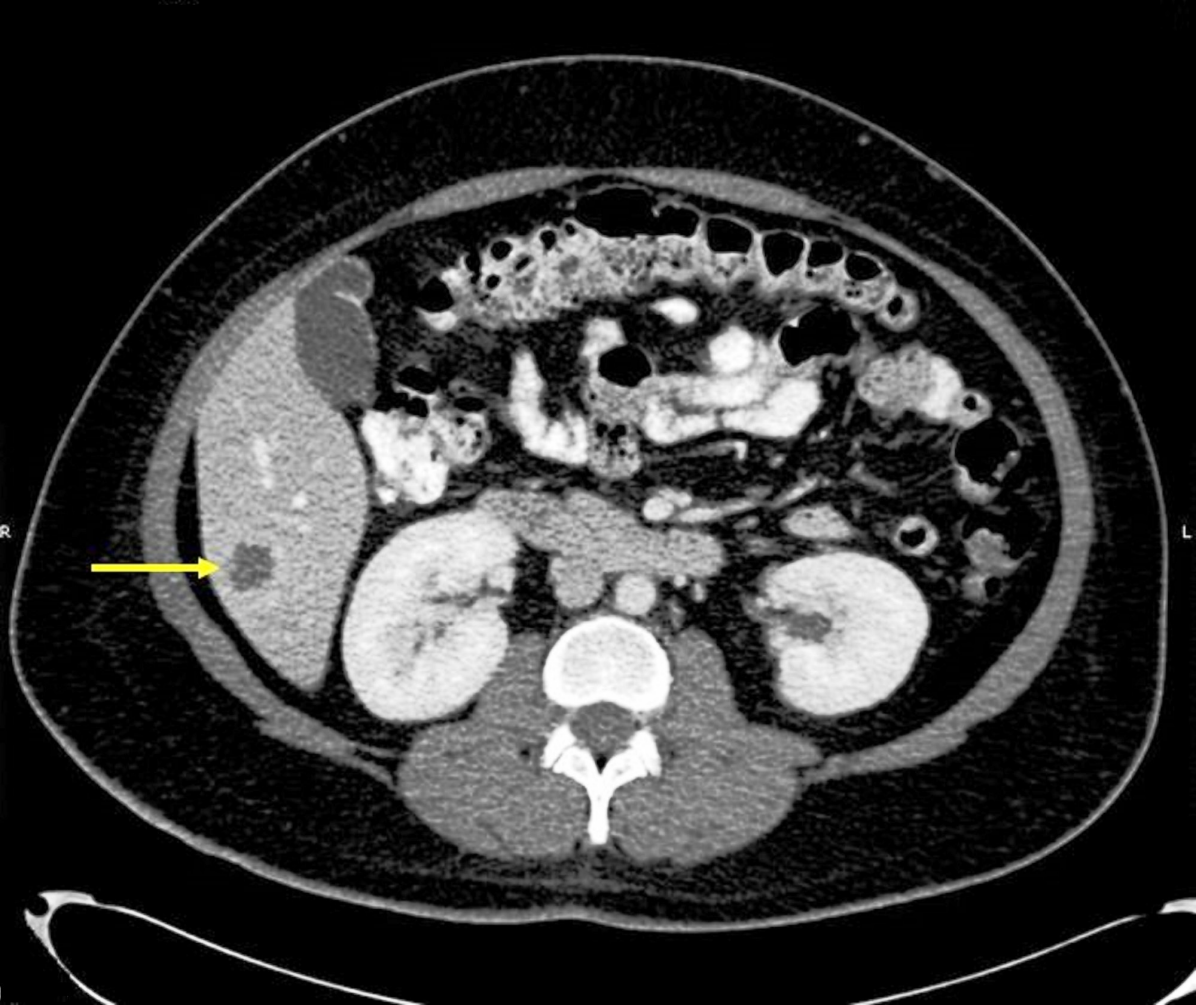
CT of the abdomen with contrast in arterial phase A hepatic segment VI 2.9 x 2.6 x 2.8 cm lesion demonstrating strong peripheral arterial enhancement with central isodense nature, which raised a concern for cystic change. However, all liver tumors get 100% of their blood supply from the hepatic artery, so when they enhance, it will be in the arterial phase.
